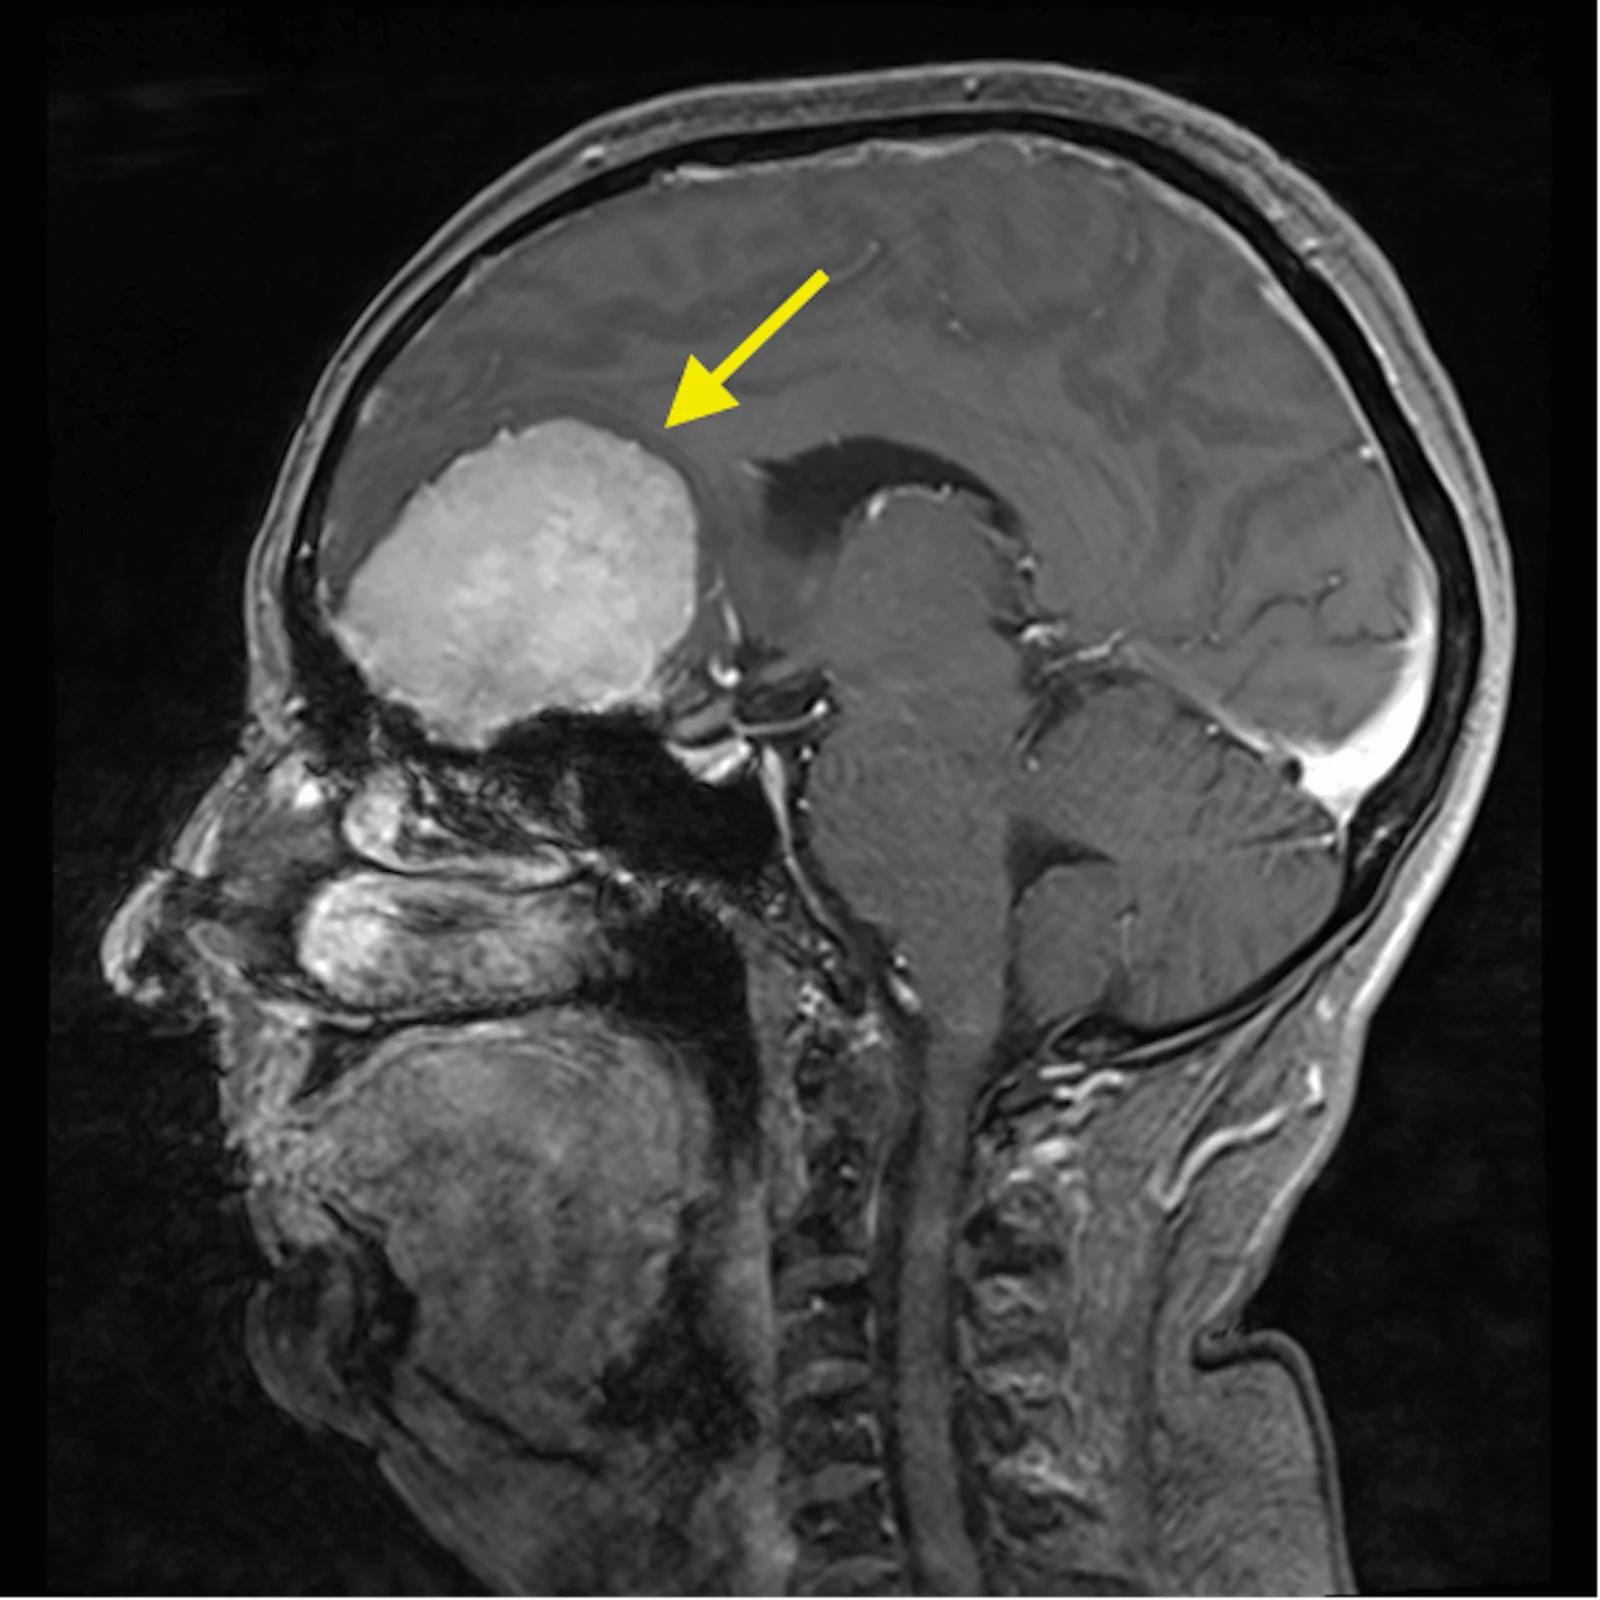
Preoperative post-contrast sagittal T1-weighted brain MRI A large, well-circumscribed, lobulated extra-axial mass centered on the olfactory groove demonstrates avid, predominantly homogeneous enhancement. The lesion causes marked mass effect on the frontal lobes and compression of the adjacent ventricular system. The solid arrow indicates the tumor MRI: magnetic resonance imaging
